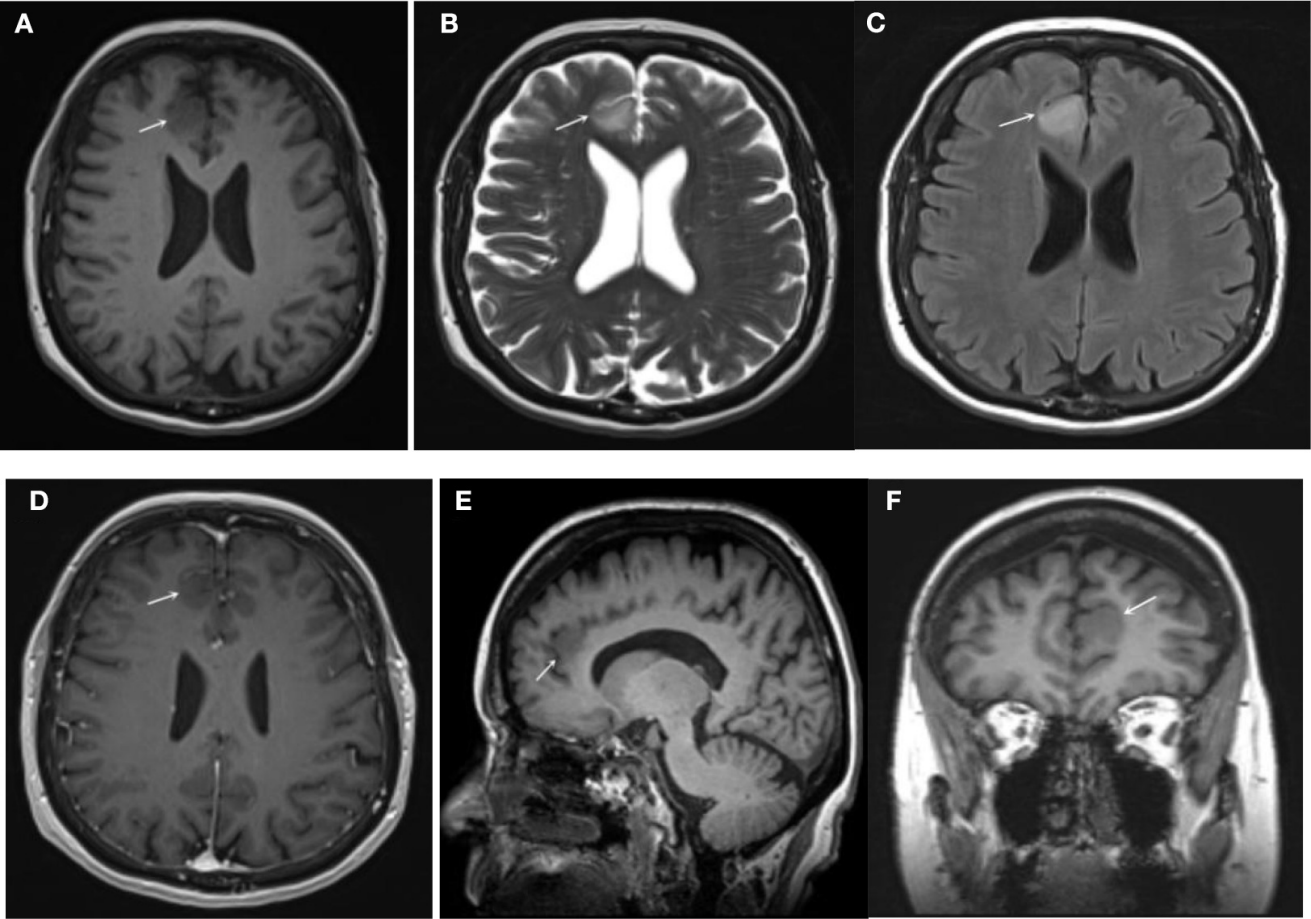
IDH1 mutated glioma (low grade): (A) T1WI (B) T2WI (C) FLAIR (D) T1+C (axial) (E) T1+C (sagittal) (F) T1+C (coronal). The lesion had a clear border, homogeneous signal intensities on T1WI and T2WI, as well as on FLAIR. The lesion presented mild homogeneous enhancement on post-contrast T1WI.
